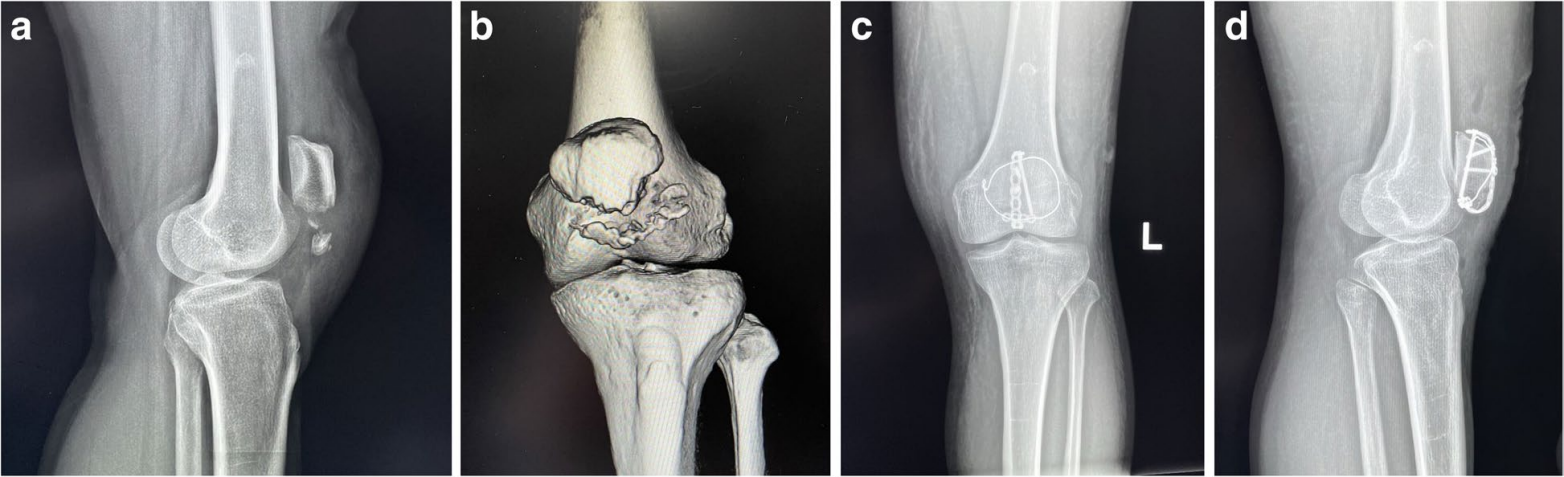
A 46-year-old female patient with distal patellar fracture. a-b Preoperative three-dimensional CT and lateral X-ray films of the knee joint; c-d anteroposterior and lateral X-ray films of the knee joint of the affected limb on the second day after the operation
